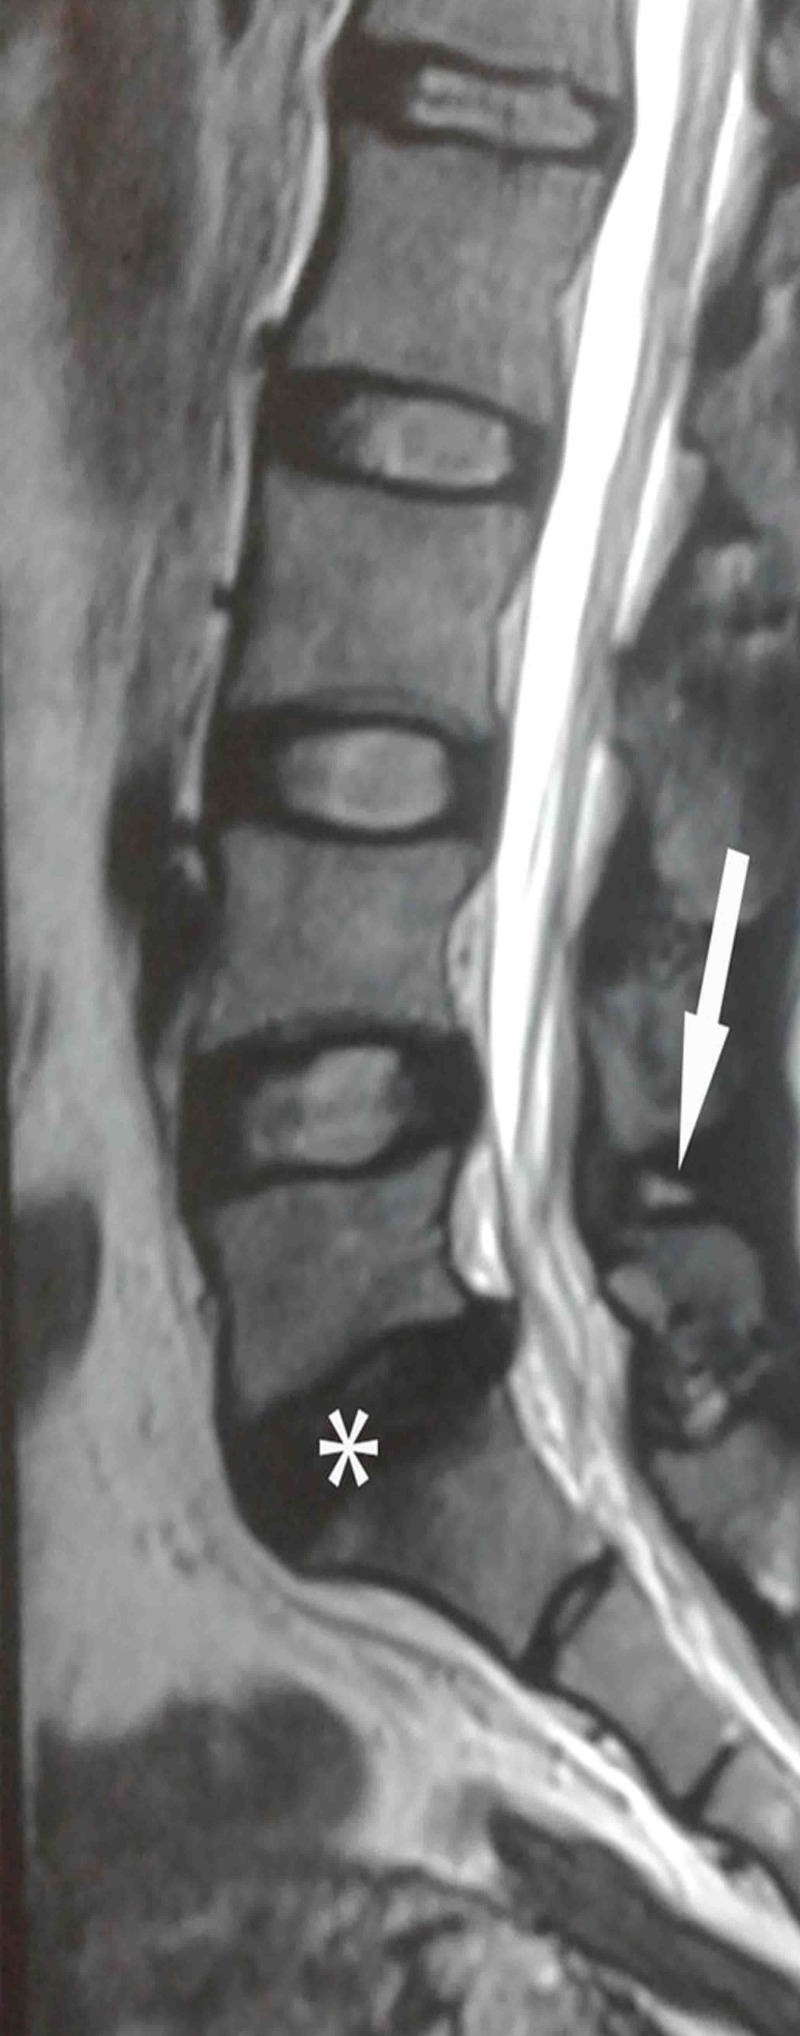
Magnetic resonance imaging (MRI) of lumbosacral spine. On longitudinal mid-sagittal scanning of the lumbosacral spine, T2-image depicts intervertebral disc degeneration and herniation between L5 and S1 vertebral bodies (asterisk) and inter-spinous ligament degeneration (high intensity on T2 image) (white arrow).
